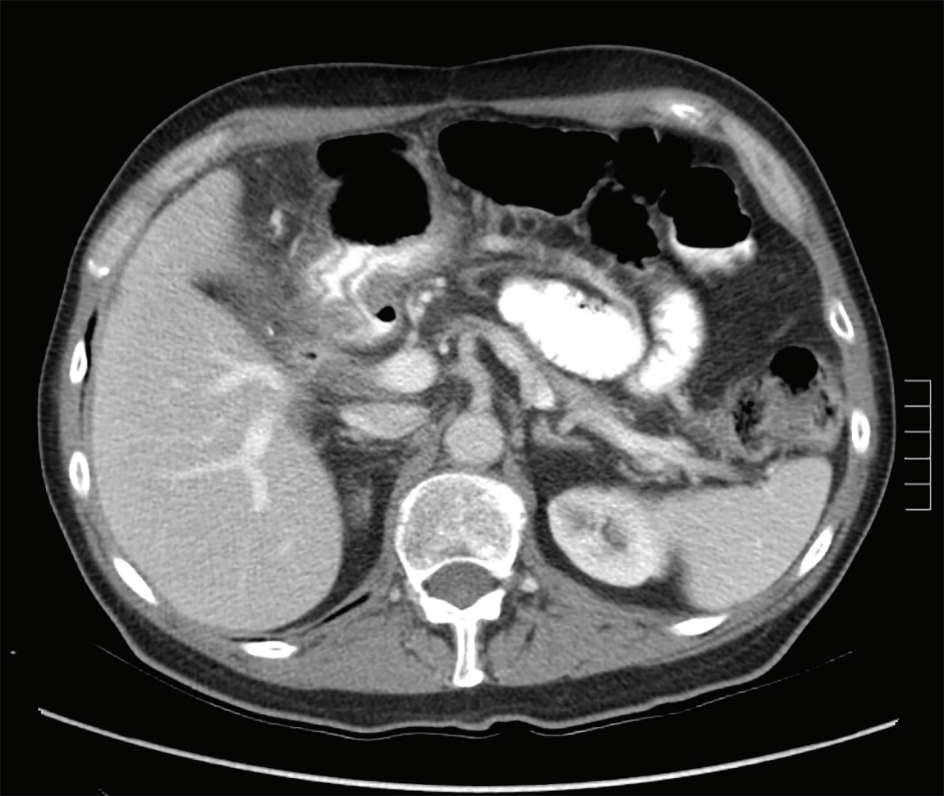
CT scan for evaluation of abdominal pain 8 years after diagnosis of pancreatic cancer demonstrating functional afferent limb syndrome.
